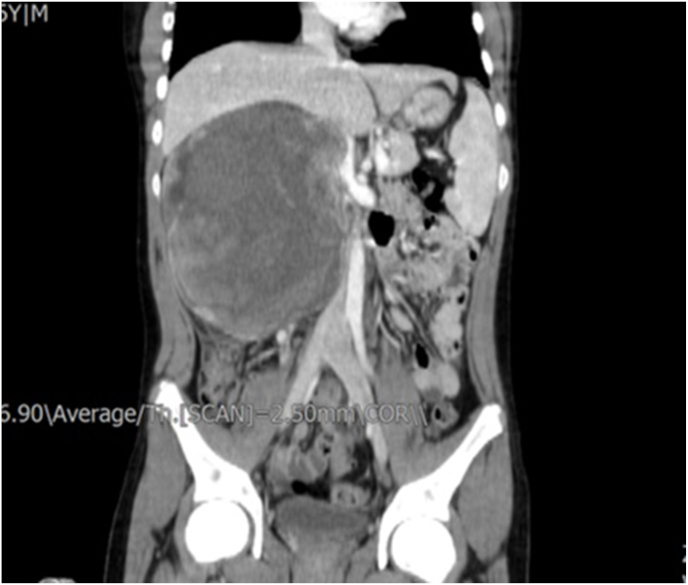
Abdominopelvic CT scan CT scan showed a retroperitoneal mass in size of 250 × 140 × 100 mm.
